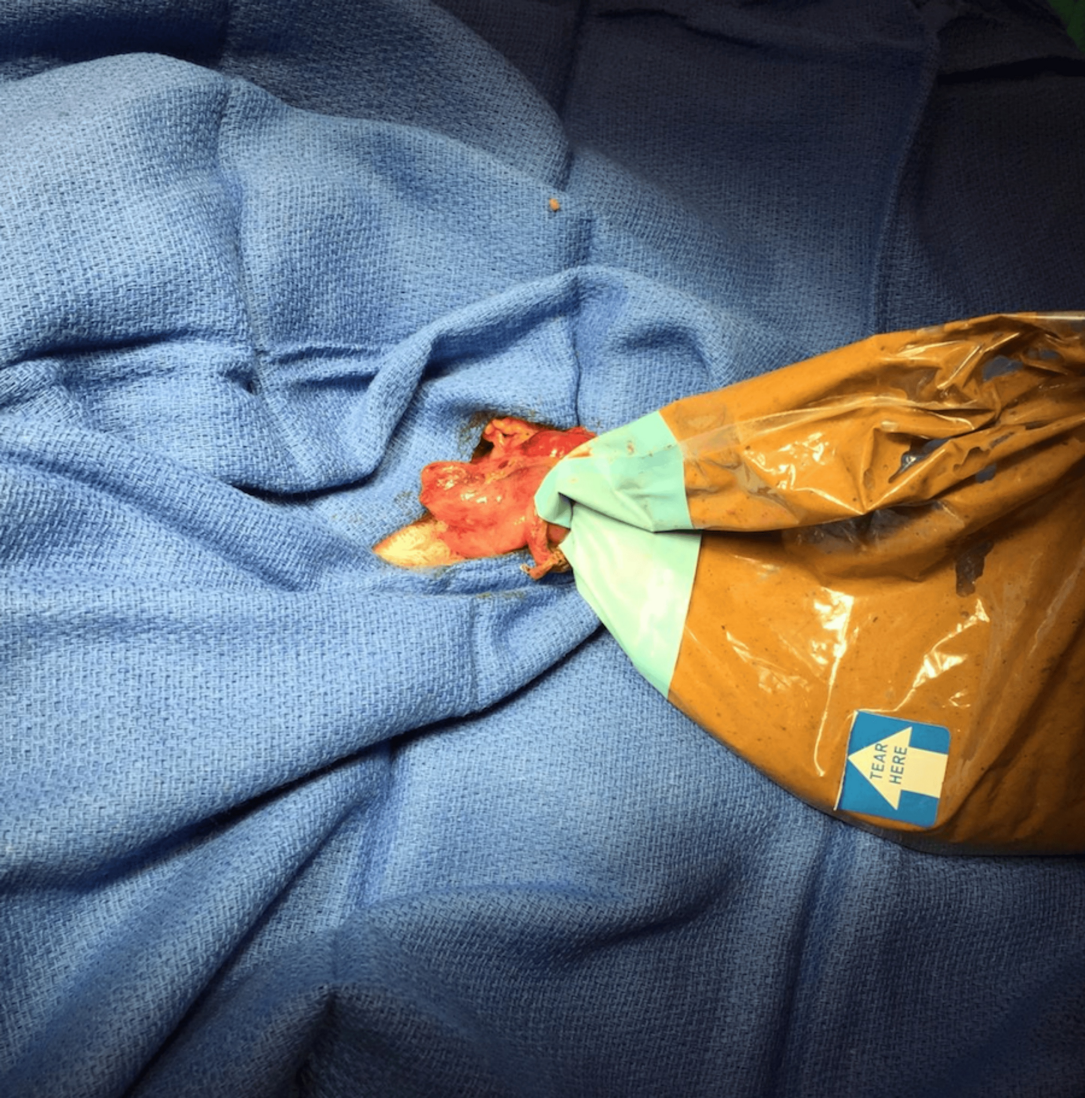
Intraoperative image showing a side-to-side anastomosis made between the descending colon and a sterile US probe sleeve US: Ultrasound
